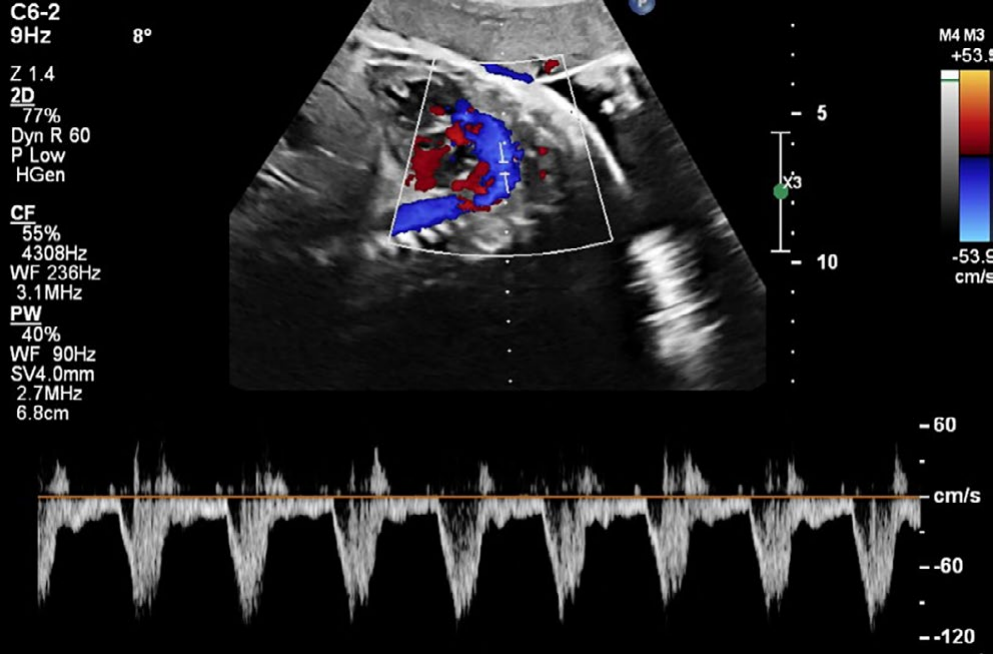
Spectral Doppler evaluation of the ductus arteriosus demonstrates decreased flow velocity, indicative of a significant reduction in stenosis severity.
